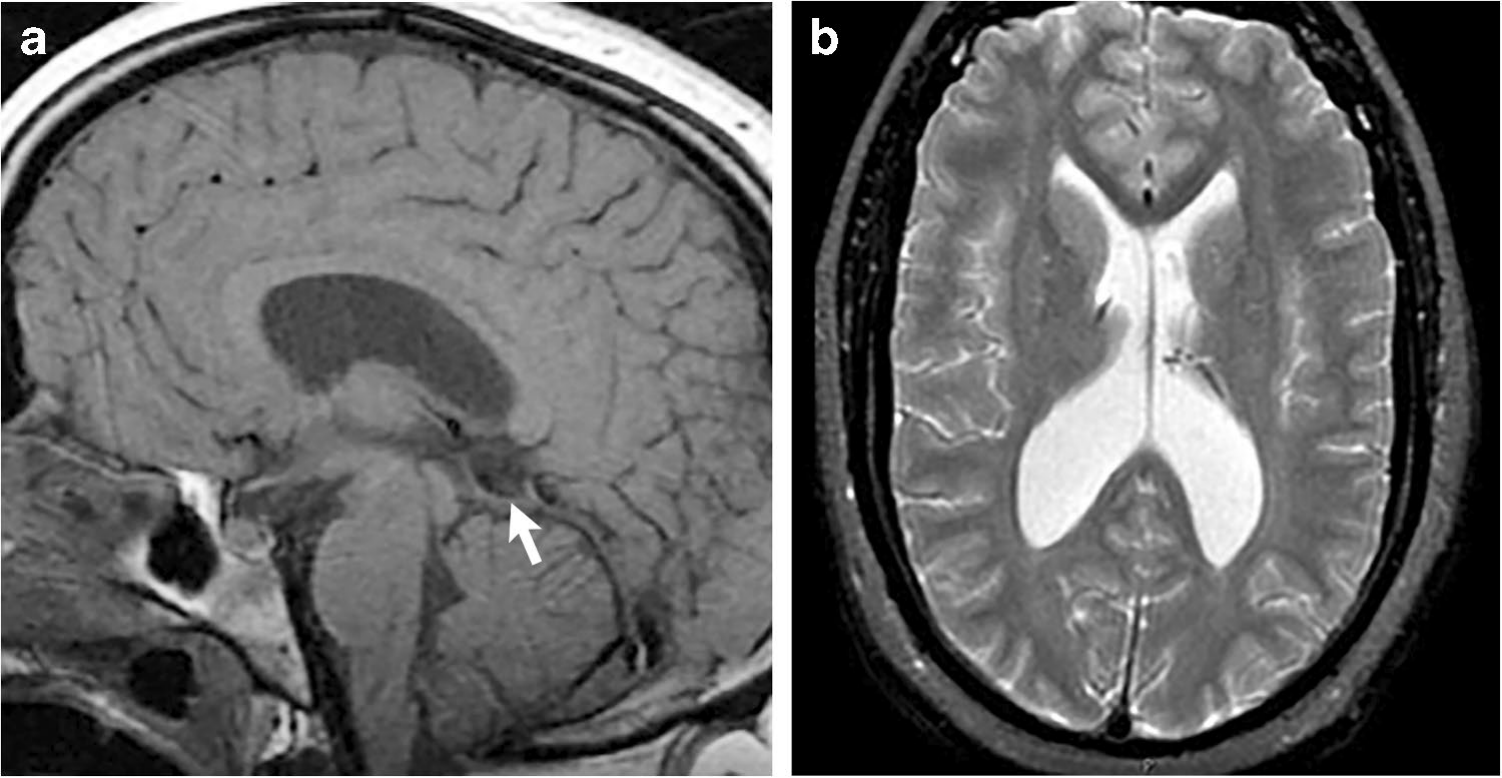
Sotos syndrome in a 17-year-old-girl with developmental delay. a A sagittal T1-weighted image shows a midline cyst (*arrow*) superior to the cerebellar vermis. b An axial T2-weighted image shows ventriculomegaly with large trigones
